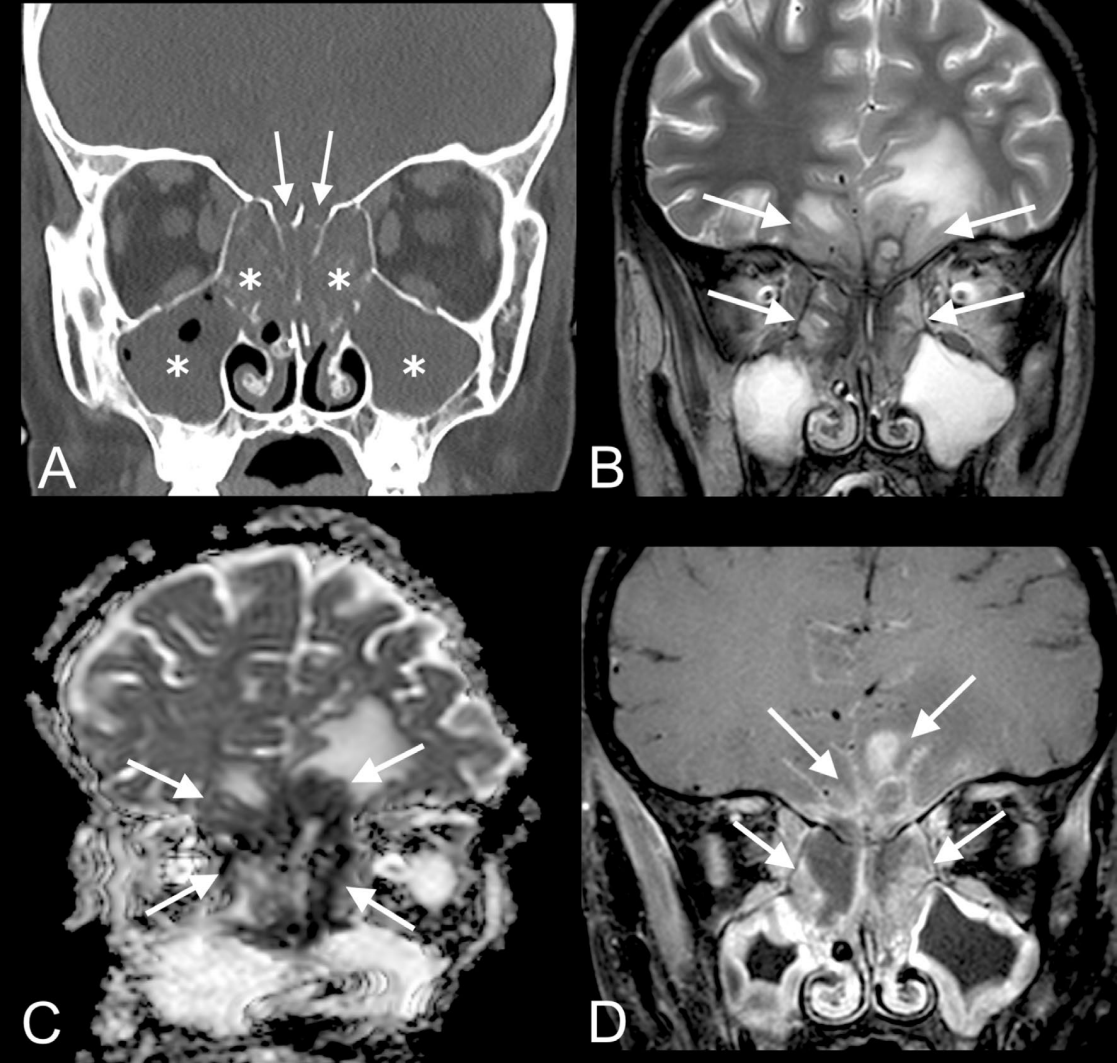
CT and MRI images of the paranasal sinuses, 9 months posttransplant. Coronal CT sinus (A) shows diffuse opacification of the maxillary and ethmoid sinuses (asterisks) with erosion of the ethmoid septations and cribriform plates (arrows). Coronal T2‐weighted brain MRI (B) shows mixed hypo‐ and hyperintense lesions (arrows) within the ethmoid sinuses that extend through the cribriform plates into the inferior frontal lobes, greater on the left than on the right, with adjacent vasogenic edema. The lesions demonstrate corresponding restricted diffusion and enhancement on coronal ADC map (C) and coronal post‐contrast fat‐suppressed T1‐weighted imaging (D), respectively. The differential diagnosis included posttransplant lymphoproliferative disorder (PTLD) and acute invasive fungal sinusitis. Biopsy of the lesion in the right sphenoethmoidal recess demonstrated monomorphic PTLD presenting as EBV‐positive diffuse large B‐cell lymphoma.
